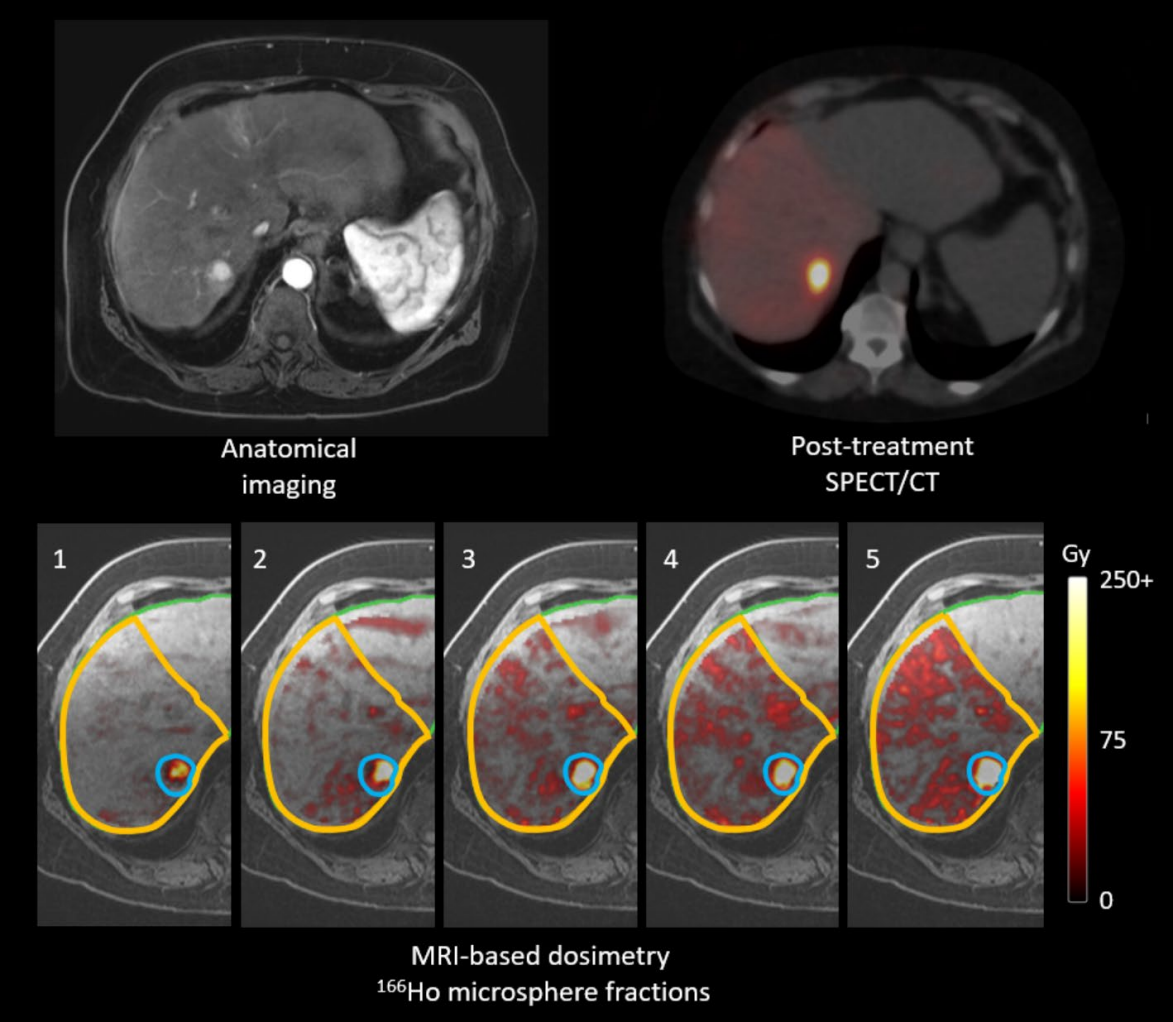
Study patient 2 with pre-treatment contrast-enhanced MRI, showing a lesion in segment 7. Post treatment ^166^Ho SPECT/CT acquired five days after treatment shows high uptake in the lesion and low homogeneous uptake in the treated non-tumoural liver. MRI-based dose maps after each given microsphere fraction using the CAD. The treated liver part is delineated, within the treated liver part the tumour is delineated. Each fraction represents an increase of 20% of the planned dose administration. The tumour dose increases with each given fraction, as well as the non-tumoural liver tissue
